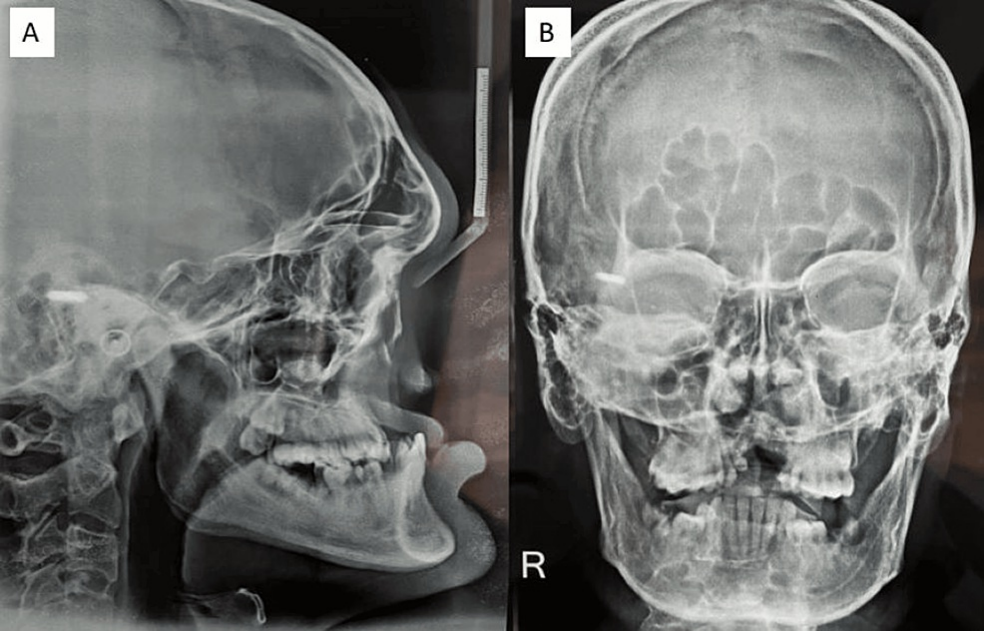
Pre-treatment radiographs: (A) Lateral cephalogram, (B) posteroanterior (PA) cephalogram.
